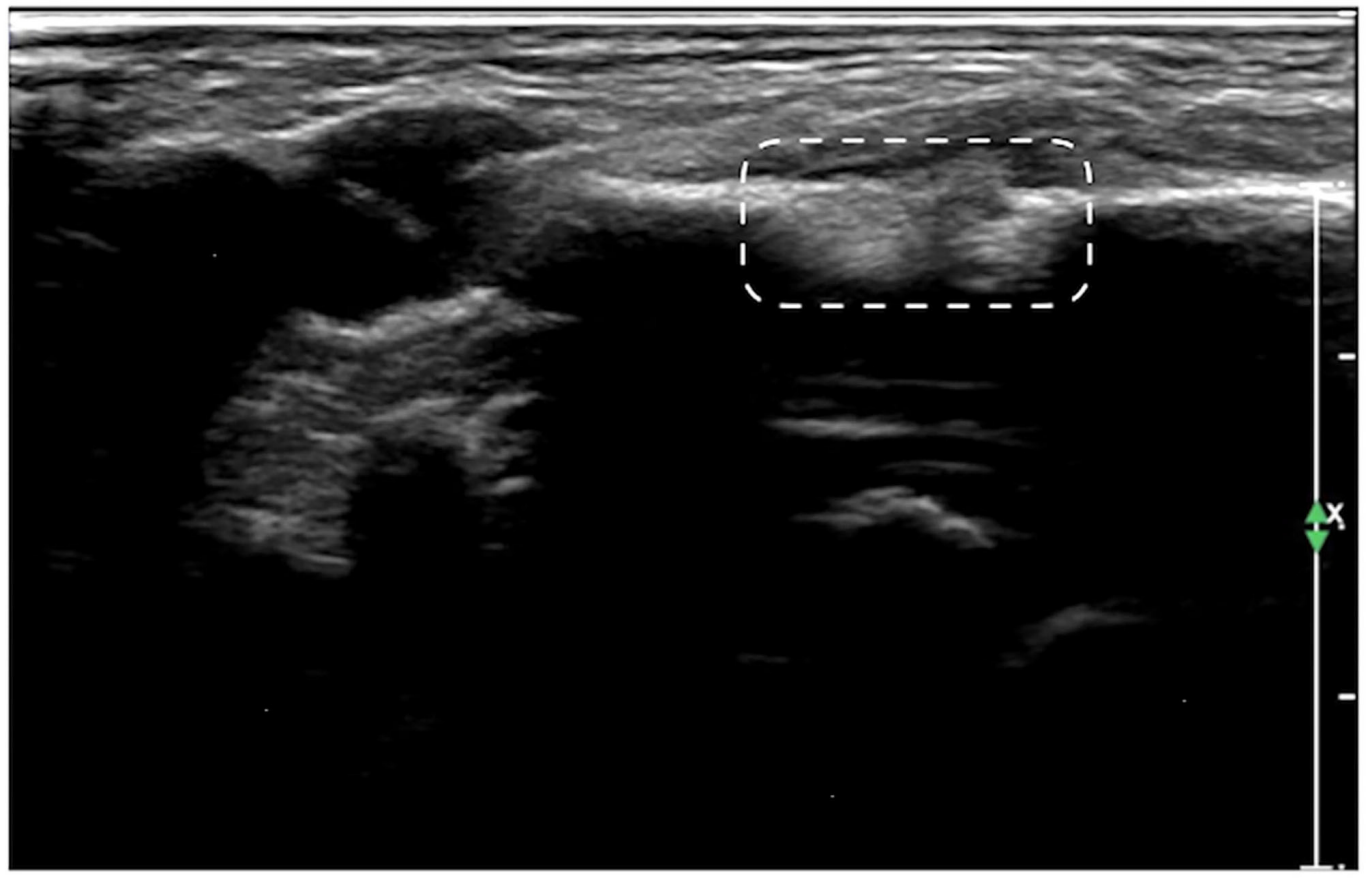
Callus formation. The infant was G_1_P_1_, with a gestational age of 39^+5^ weeks, vaginal delivery, and a birth weight of 3,700 g. The infant was admitted to the hospital on the 7th day after birth due to jaundice. Physical examination revealed local swelling and callus formation on the medial segment of the right clavicle. Ultrasound examination showed interrupted continuity and local bone tissue proliferation forming a callus at this fracture site (within the dashed line).
